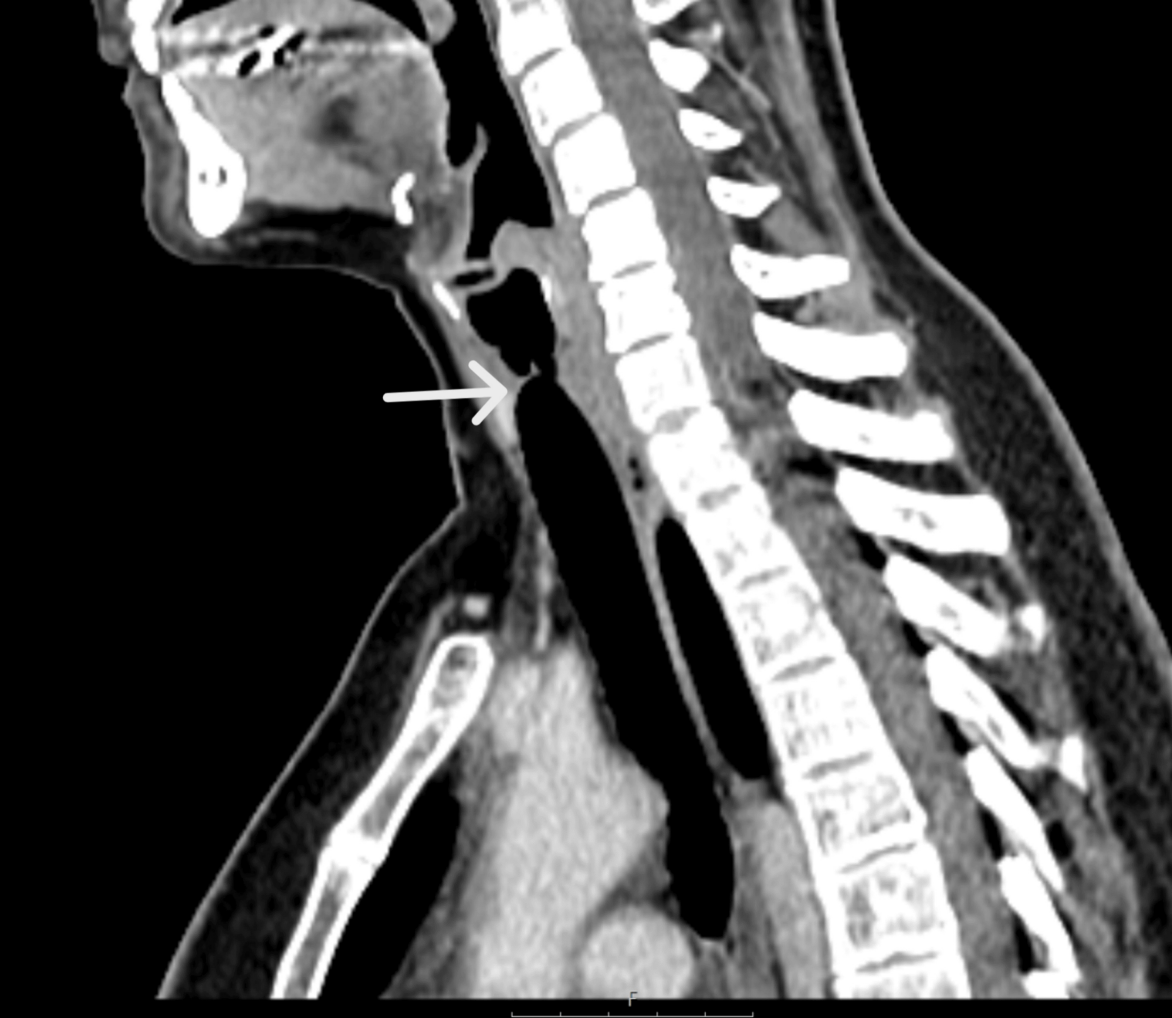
Sagittal CT showing short-segment stenosis
